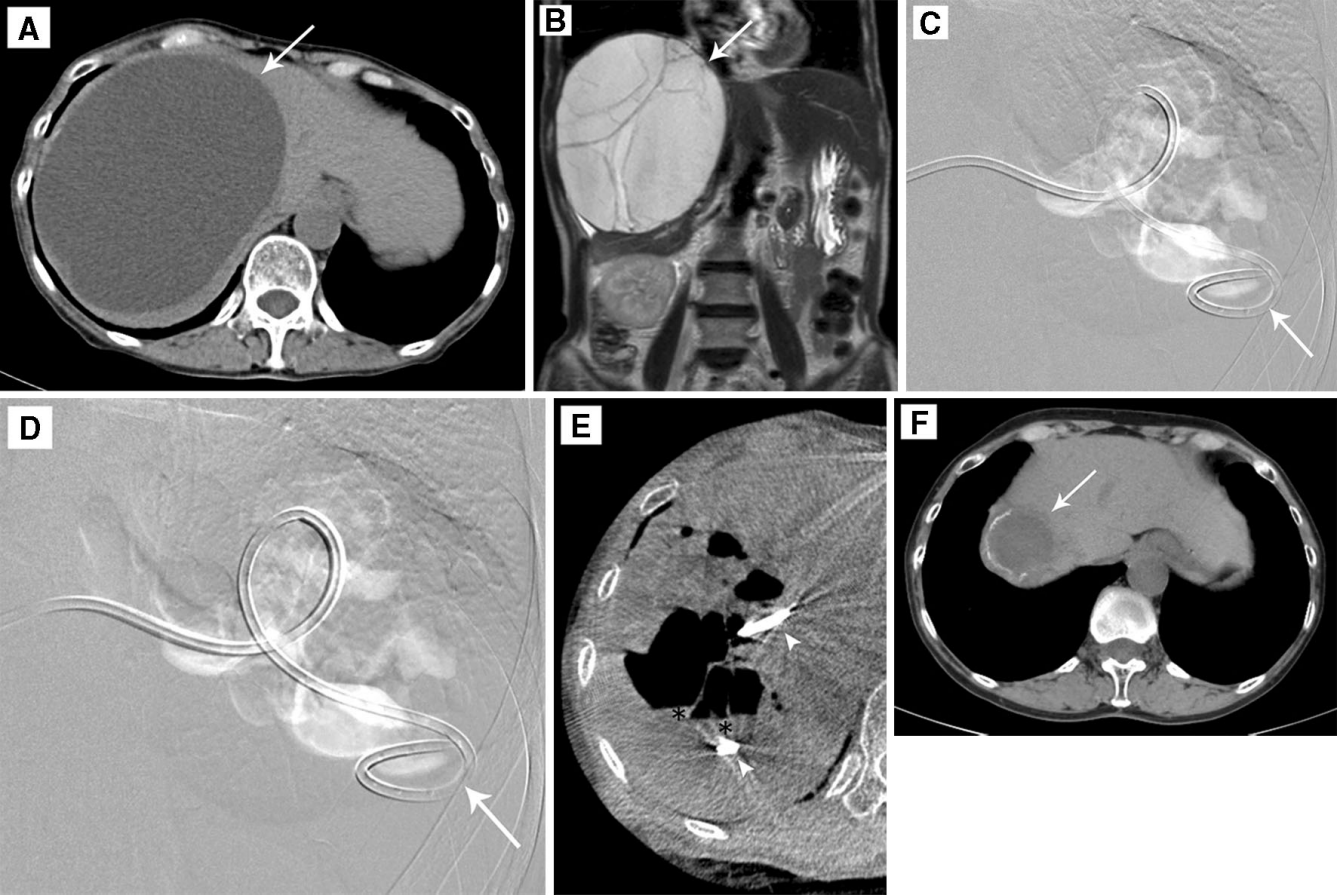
A 67-year-old woman (Case 1) with a symptomatic hepatic cyst causing abdominal distension. CT scan (*arrow*, A) shows a huge cyst in the right lobe of the liver, which is depicted as a well-demarcated cystic mass with several septums projecting to the right diaphragm on a coronal T2-weighted magnetic resonance image (*arrow*, B). While the patient was in a prone position, 10 ml of polidocanol foam was injected under fluoroscopy (D) through a pigtail catheter (*arrow*, C, D) placed in the cyst. This was performed after digital subtraction cystography (C) with 10 ml of CO_2_ in the same position. The distribution of the foam sclerosant was almost identical to that of CO_2,_ without any evidence of migration to the systemic circulation or surrounding hepatic vessels. E Axial reformation C-arm CT images depict the foam sclerosant as air density area distributed on the upper side within the cyst without any mixture or dilution and with a small amount of residual cyst fluid (*asterisk*) after percutaneous aspiration in the supine position. Note the pigtail catheter in the targeted cyst (*arrowheads*). F CT performed 6 months after sclerotherapy showing that the cyst was remarkably reduced in size (*arrow*)
